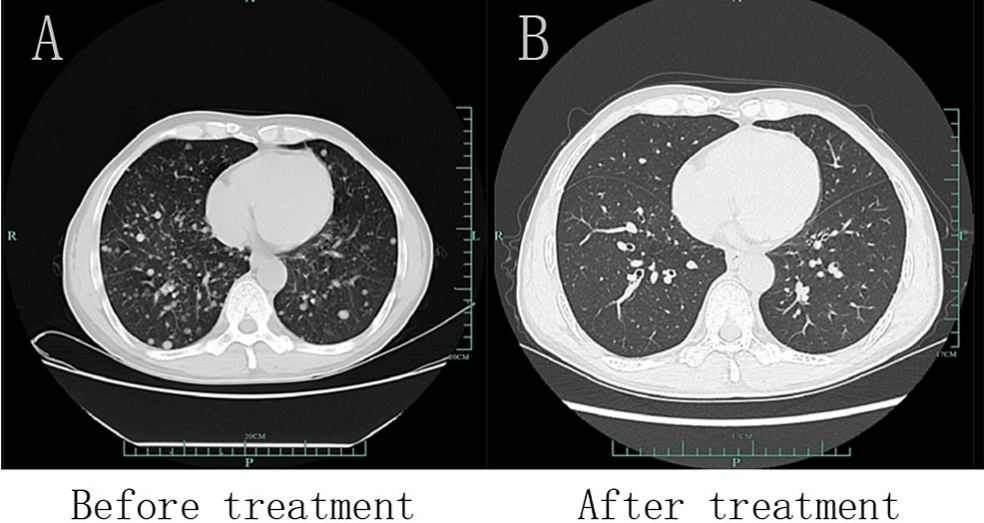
After TACE treatment with targeted immunotherapy, the metastatic lesions in the lungs had almost completely disappeared, with only one remaining in the left lung. A. CT image of the lung before treatment; B. CT image of the lung after treatment. TACE: transarterial chemoembolization
